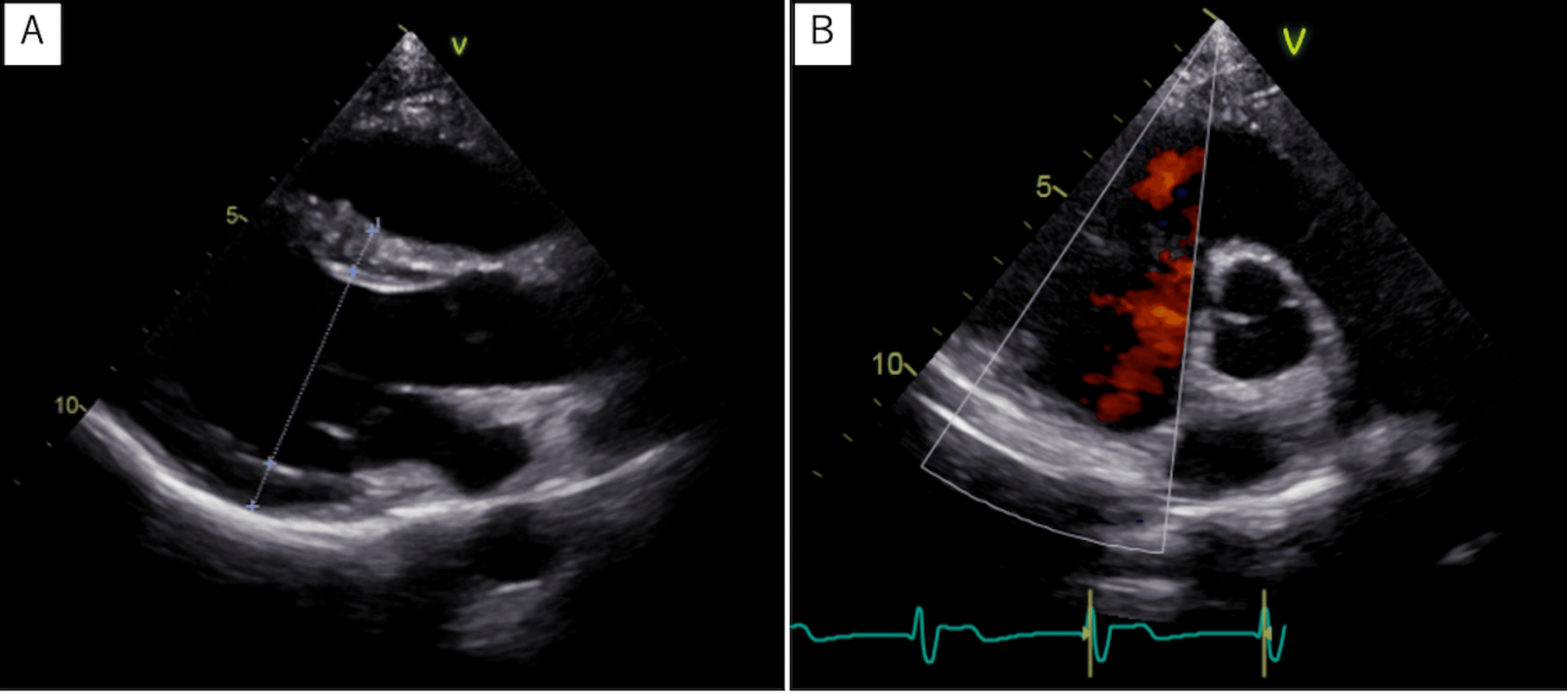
Postoperative echocardiographic view of the tumour in the right ventricular outflow tract A parasternal long axis tomogram (A) and a short-axis tomogram of the aortic valve level (B) show no residual tumor.
